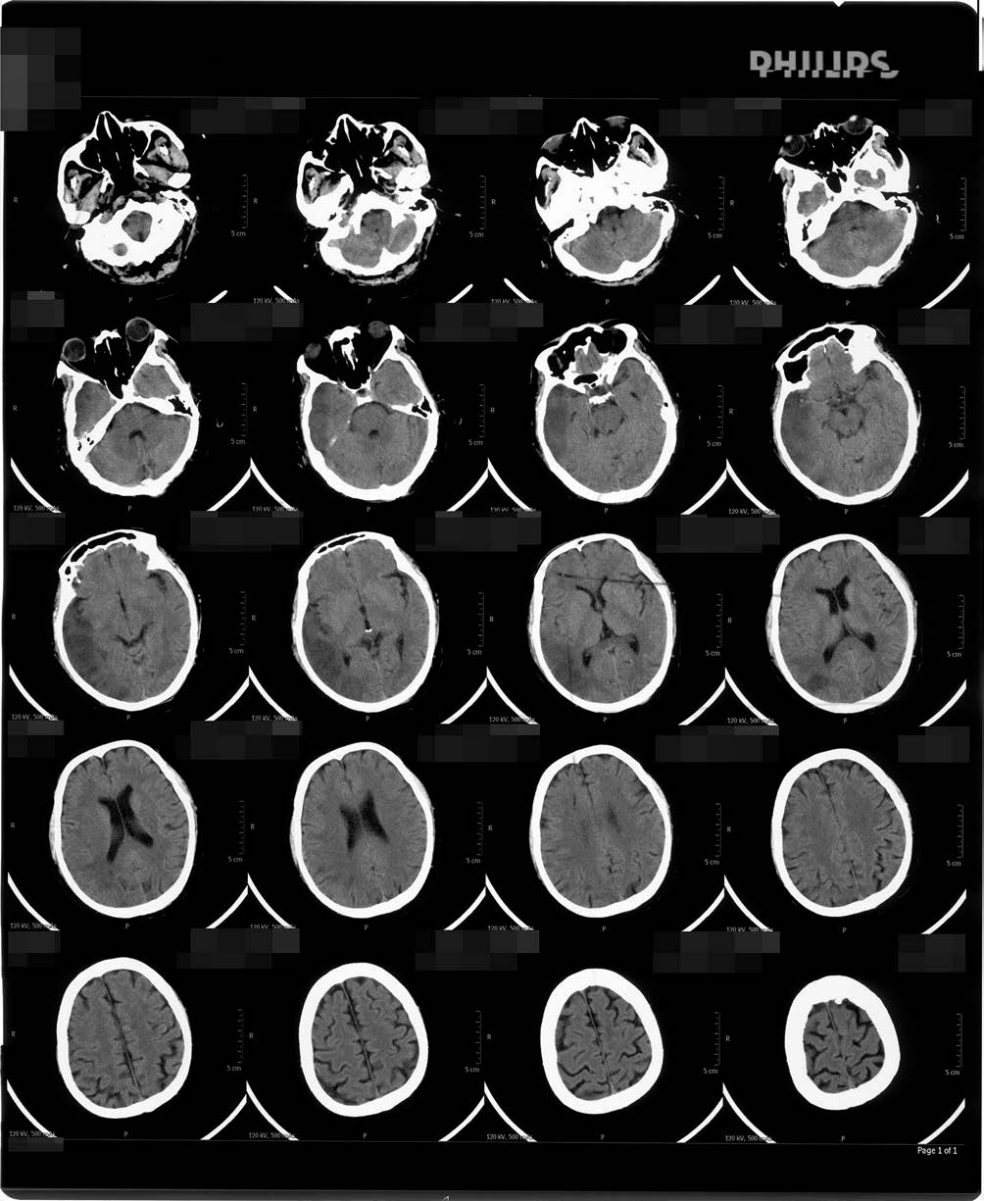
Cranial computed tomography imaging on May 22 showed improvement of the cerebral infarction compared with the results from May 13.
